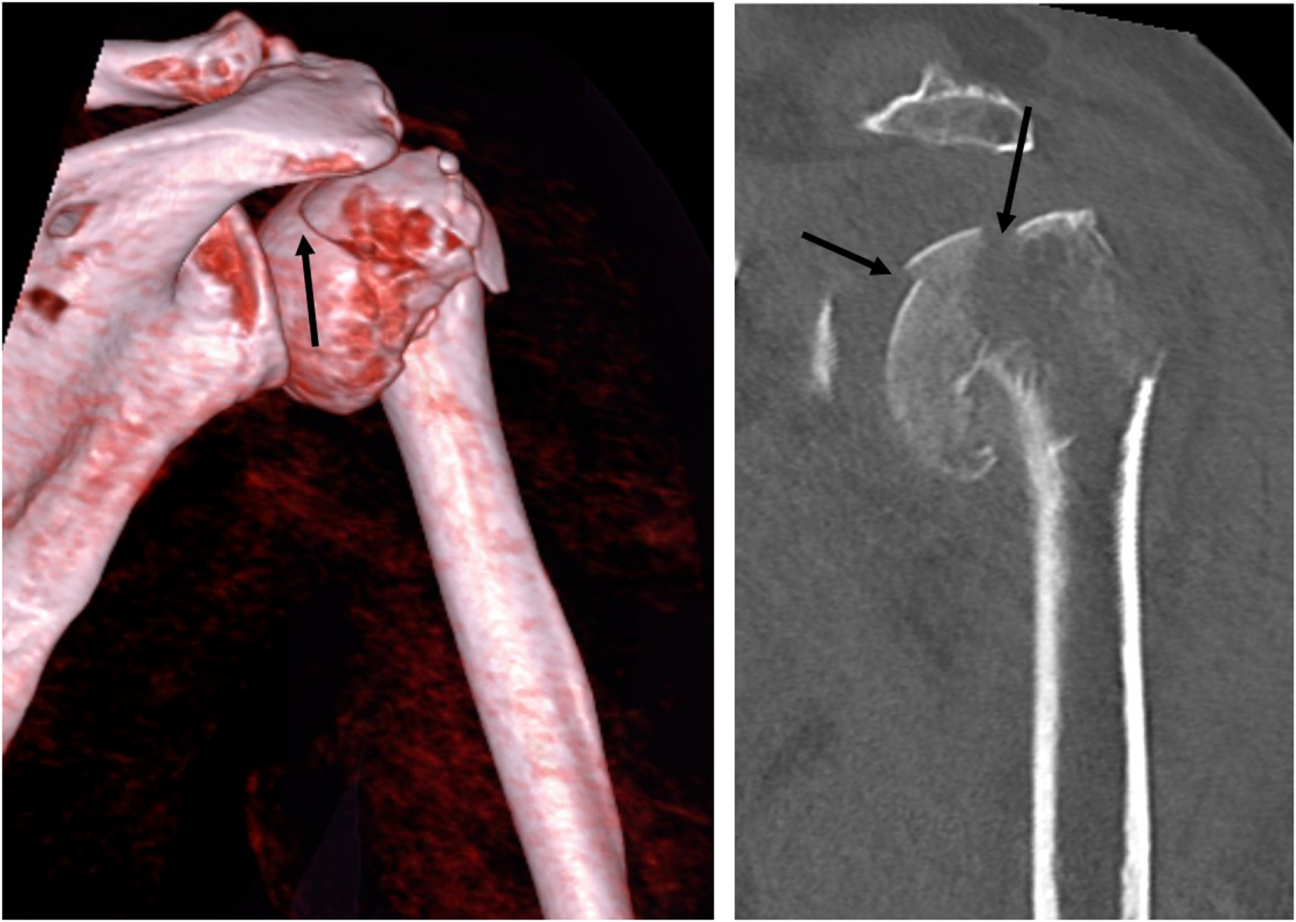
Ct images of patients with headsplit fractures included in the study: dislocated (>5 mm) fractures (arrows) located >5 mm within the cartilaginous articular surface. Left, 3D reconstruction of a CT scan; right, a coronal slice.
